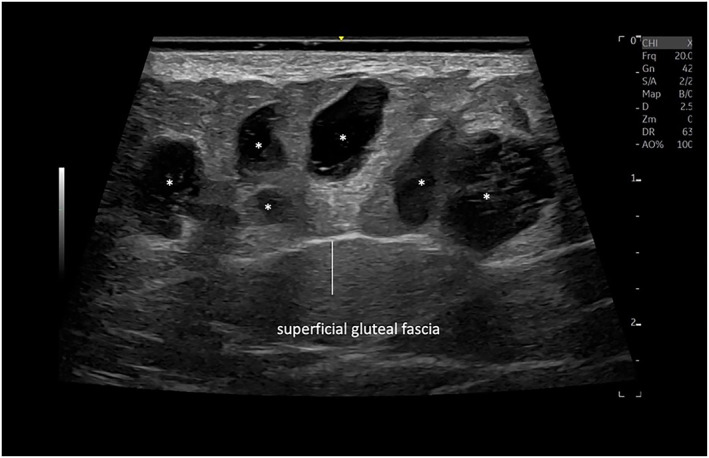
Sonographic image of the hip dips using a multifrequency linear probe ranging from 6 to 24 MHz demonstrates anechoic pseudo cystic deposits (*) above the superficial gluteal fascia of the hip dips.
